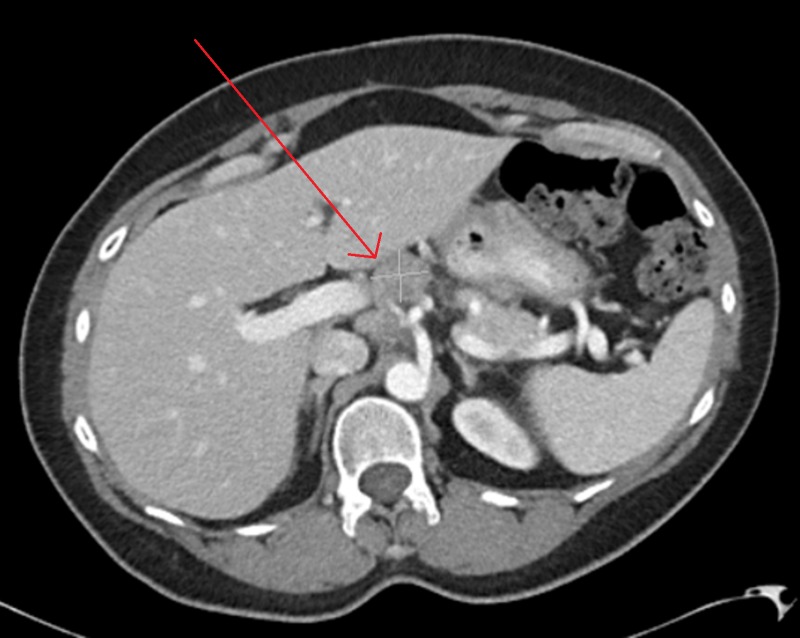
Abdominal CT of Patient 1 prior to starting evofosfamide, demonstrating a 2.5x2.8 cm target lesion, with CA-125 of 198 U/ml
